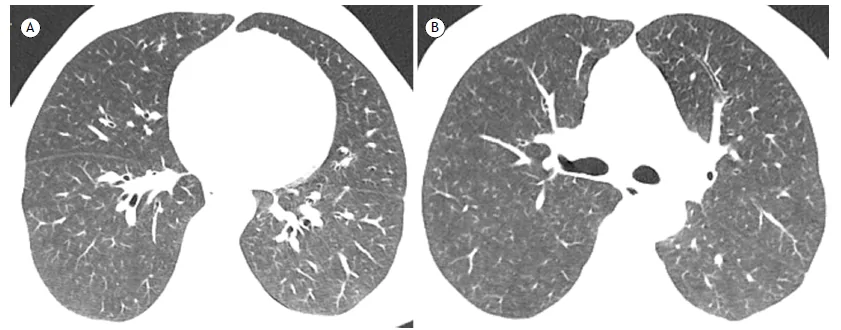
Chest HRCT scans showing bilateral diffuse centrilobular ground-glass micronodules (in A and B).
